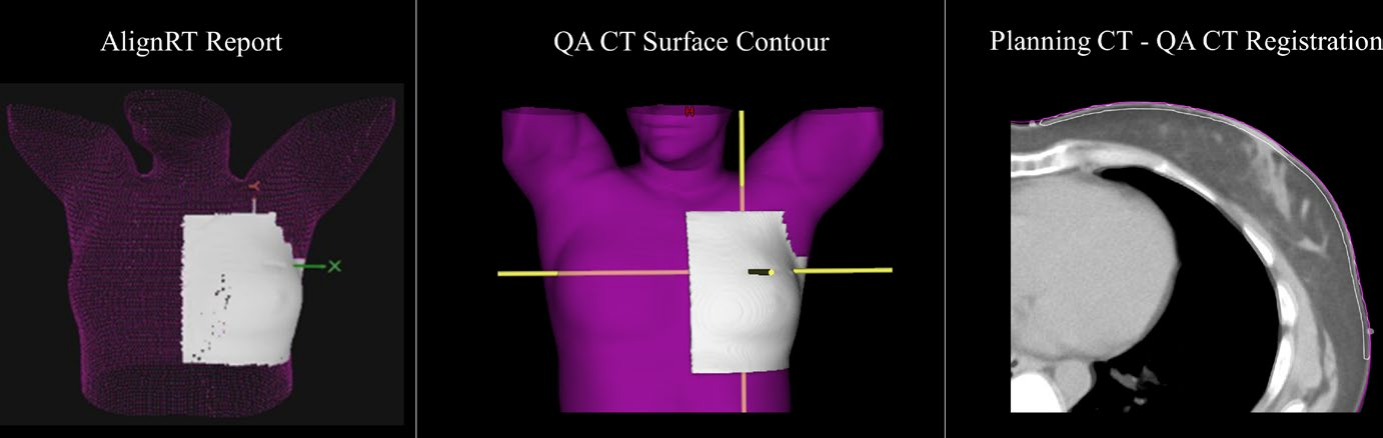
Surface‐guided radiation therapy (SGRT) setup registration. Left: The surface used for treatment setup by the AlignRT system. Middle: Recreation of the surface surveyed by the AlignRT system using a manually generated chest surface contour (shown in white) on the quality assurance CT (QA‐CT). Right: Fusion image from the planning CT and the QA‐CT after performing an automatic image registration focusing on the chest surface contour only
